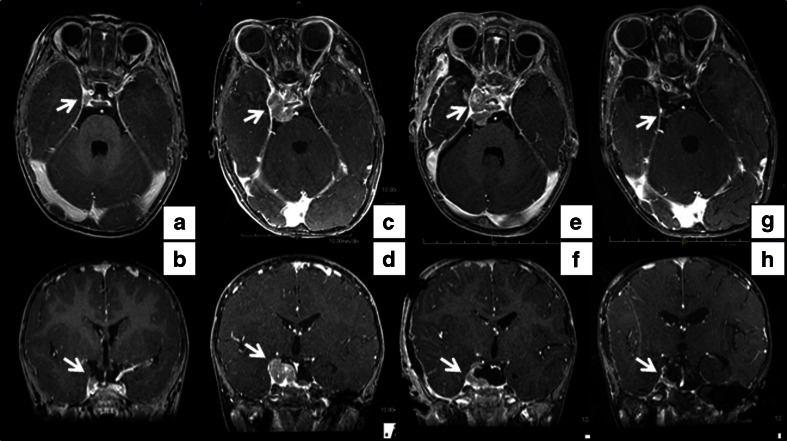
Brain MRI with gadolinium enhancement (axial and coronal sections). On admission day 1, MRI revealed a 9 × 4 mm tumor at the right cavernous sinus (a, b). Three weeks after admission, the cavernous sinus tumor had rapidly increased to 30 × 20 mm in size. The tumor invaded the cavernous sinus to the pontine cistern and compressed a part of the pons (c, d). About 50% of the tumor was resected by surgery (e, f). Six weeks after starting chemotherapy, the tumor size had reduced, and only a 7 × 4 mm tumor remained at the pontine cistern (g, h)
